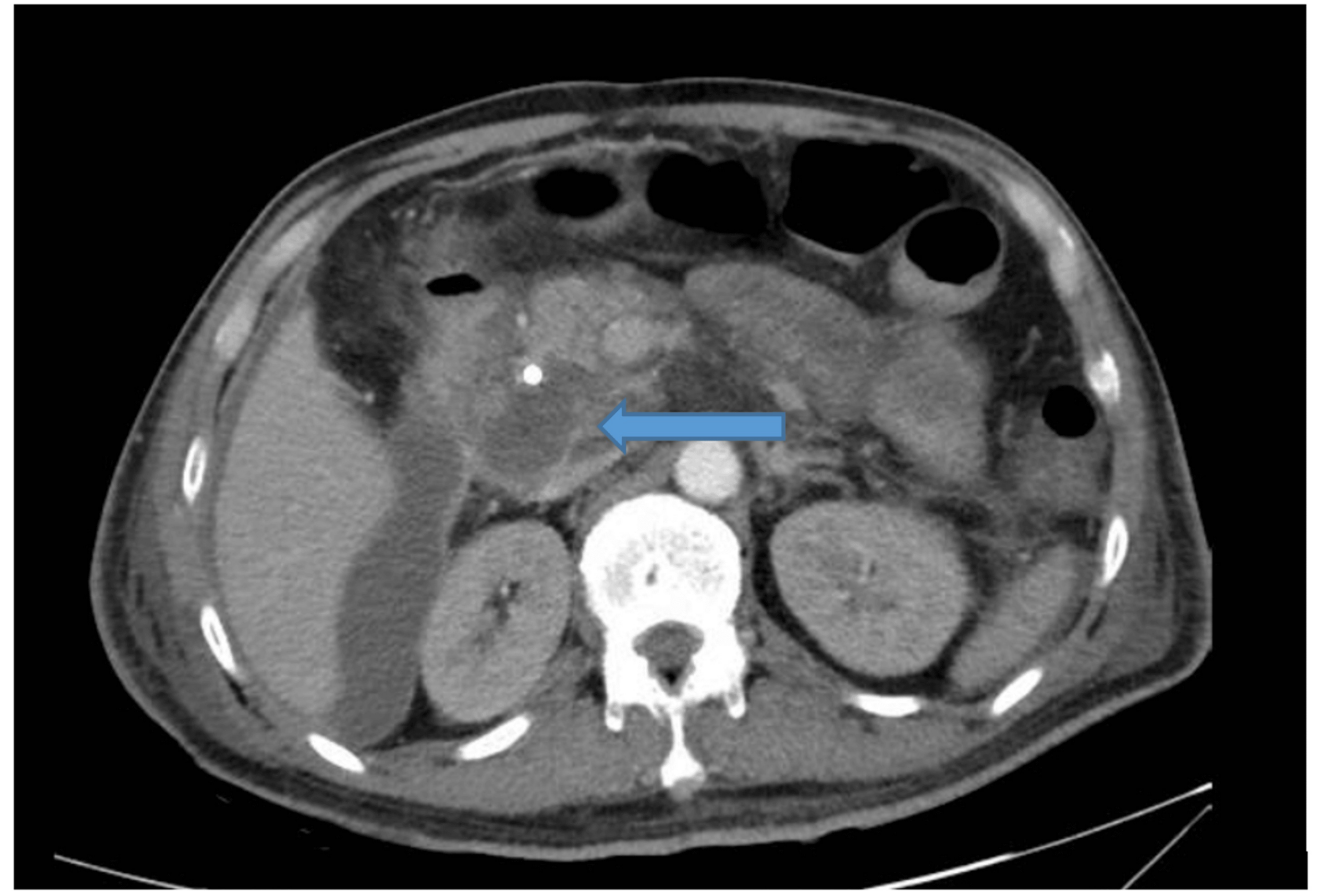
CT scan showed a persistent fluid collection (13 × 30 mm) posterior to the second and third portions of the duodenum.
